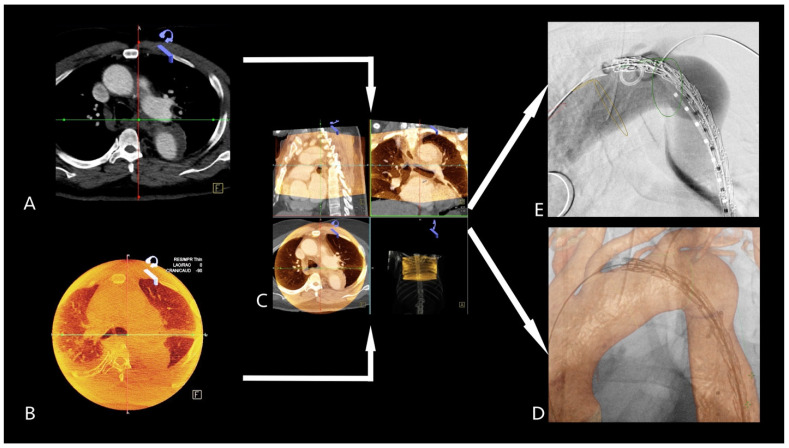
The preoperative CTA (A) and the intraoperative non-contrast CBCT (B) were used for fusion (C). The anatomically relevant structures were annotated, and the mask was overlayed on the live fluoroscopy image (D,E). Abbreviations: CTA–computed tomography, CBCT–cone-beam CT.
